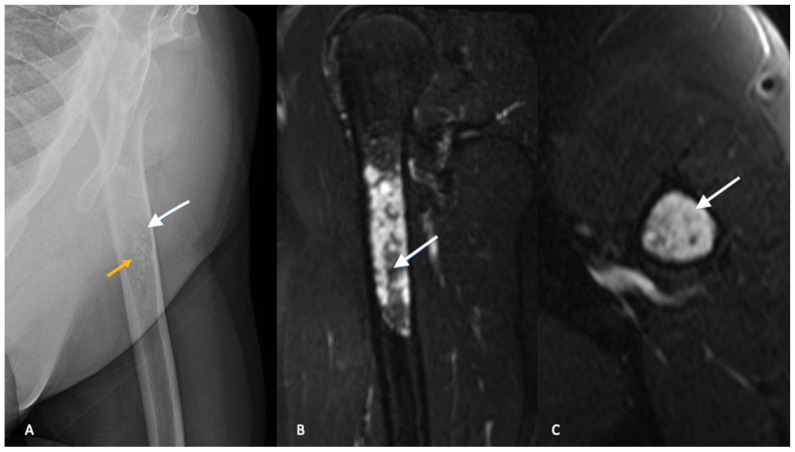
Radiograph (A) of the left proximal humerus demonstrates subtle lucency with internal stippled calcified foci (yellow arrow), in keeping with chondroid matrix. Subtle endosteal scalloping is present (white arrows) without cortical breakthrough or periosteal reaction. Sagittal (B) and axial (C) T2-weighted images with fat saturation of the same patient demonstrating a lobulated T2 hyperintense mass. A few stippled areas of low signal intensity represent chondroid matrix (white arrow). The lesion measures greater than 5 cm in length. Axial images demonstrate endosteal scalloping at the anterolateral border. No periostitis or cortical breakthrough. Imaging features are in keeping with an atypical cartilaginous tumor, which was confirmed on pathology after surgical curettage.
